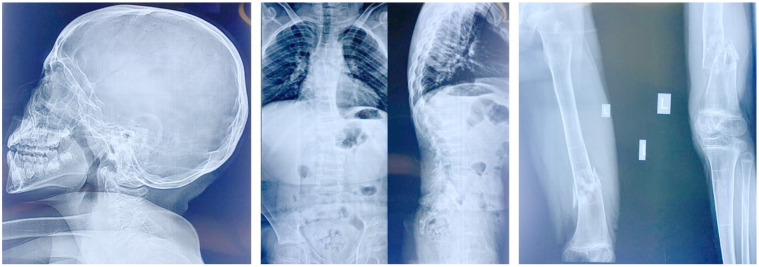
X-rays of patient 1 showing thinning of the occipital bone, abnormal vertebrae, kyphoscoliosis, femoral fractures, and bone demineralization.
